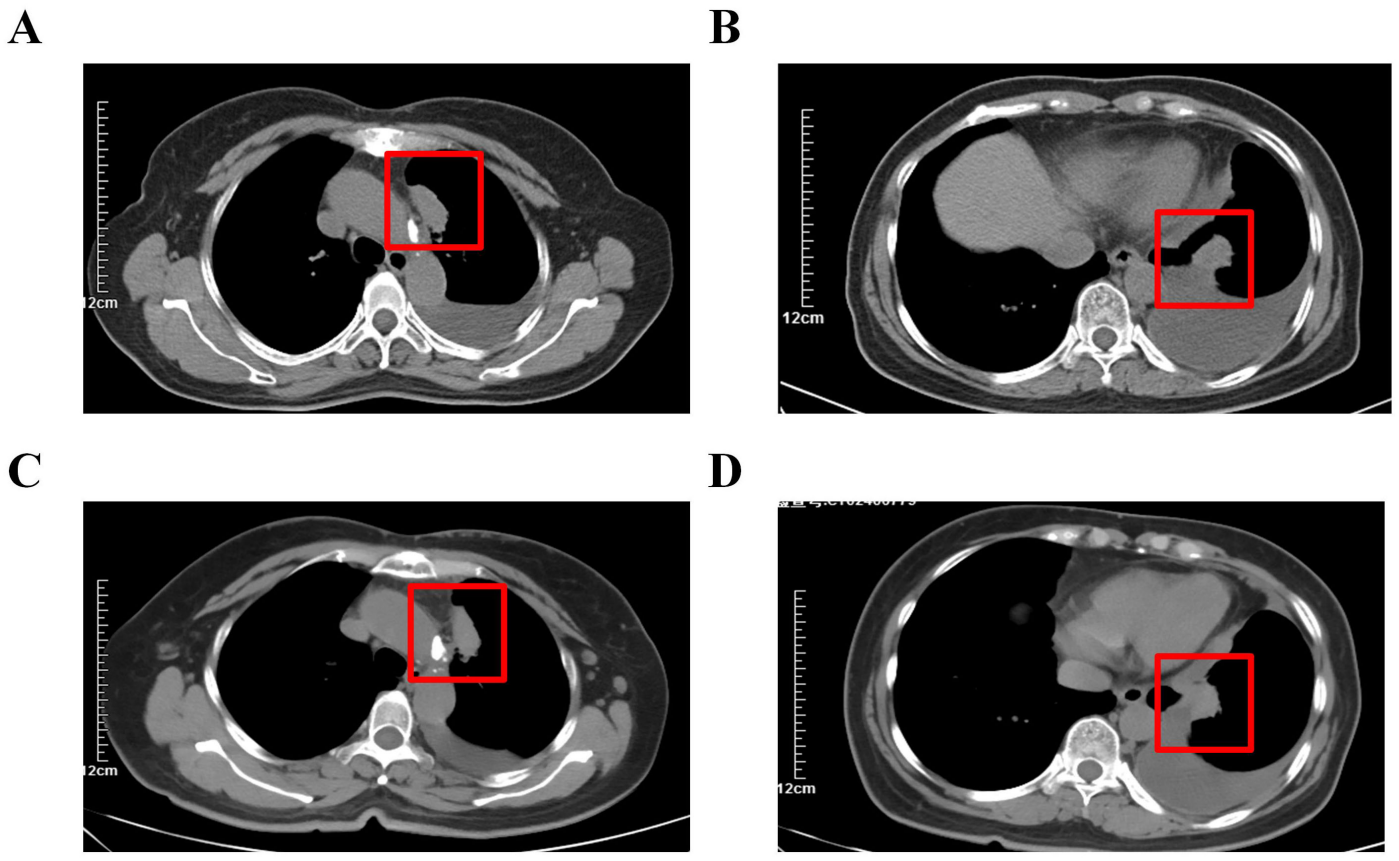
(We have updated the images to replace the previously submitted ones, ensuring that all patient information is properly concealed to protect privacy.) Changes in target lesion during treatment. (A, B) CT scan before treatment. (C, D) CT scan after 1 cycles of treatment. he red arrows mark the target lesions. The red box marks the target lesions.
